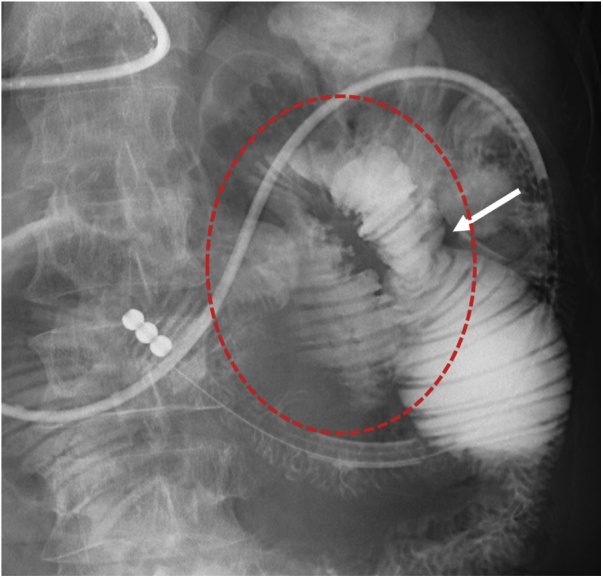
Gastrografin contrast radiography through a long tube showing the closed loop (dotted red circle) and caliber change of small intestine (white arrow).
